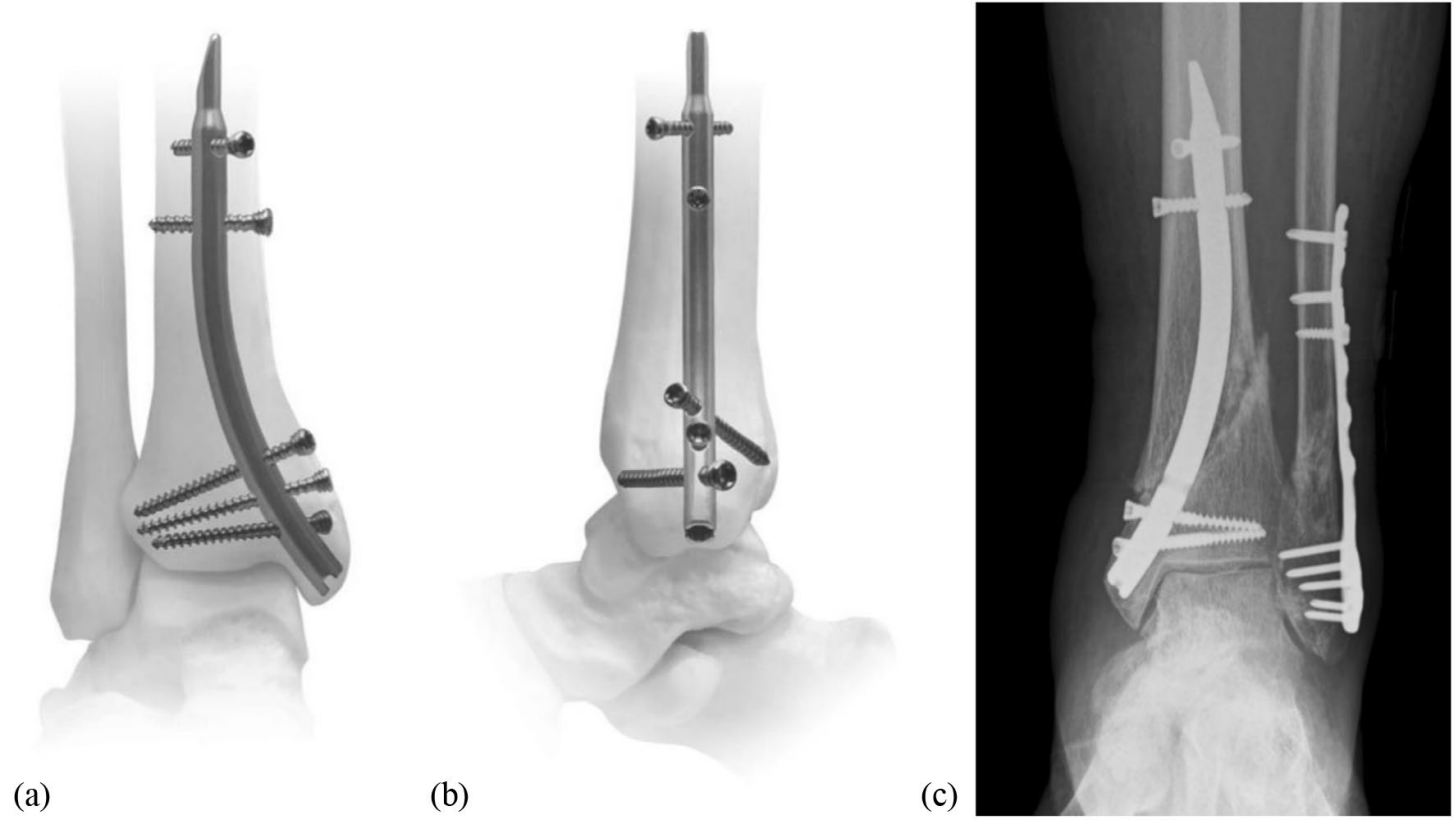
View of an implanted Distal Tibia Nail (DTN) in the antero-posterior (a) and medio-lateral (b) axes, and a patient radiography where only double-locking was possible distal to the fracture
